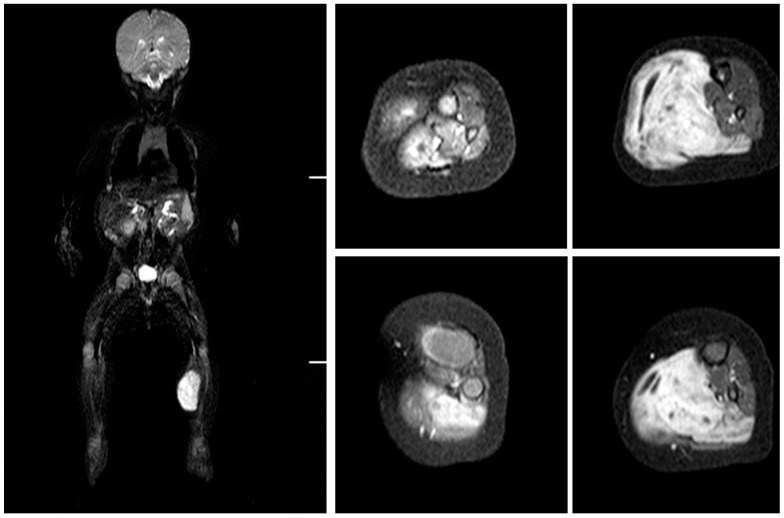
Magnetic resonance imaging (MRI) at three months old. RMS (rhabdomyosarcoma) mass in the superficial posterior compartment of the left lower limb.
